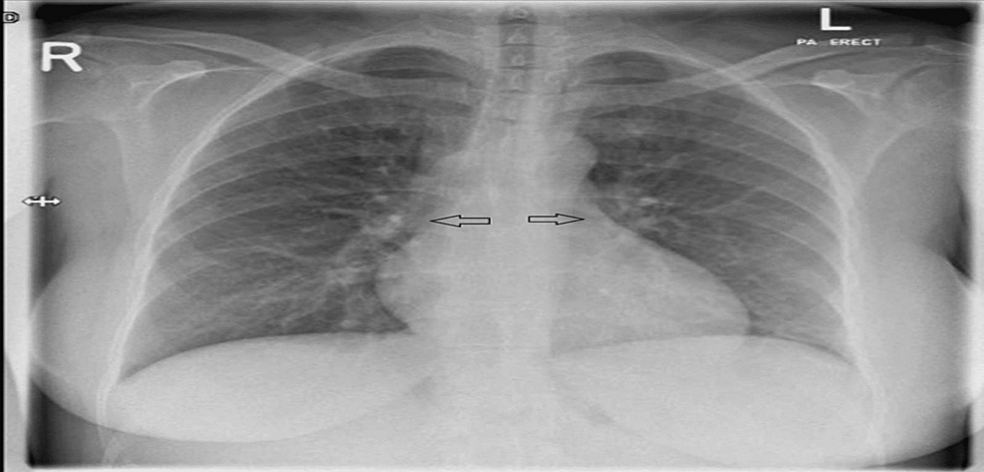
Chest X-ray arrows show bilateral prominent bronchovascular markings with no obvious consolidation or infiltrate seen
